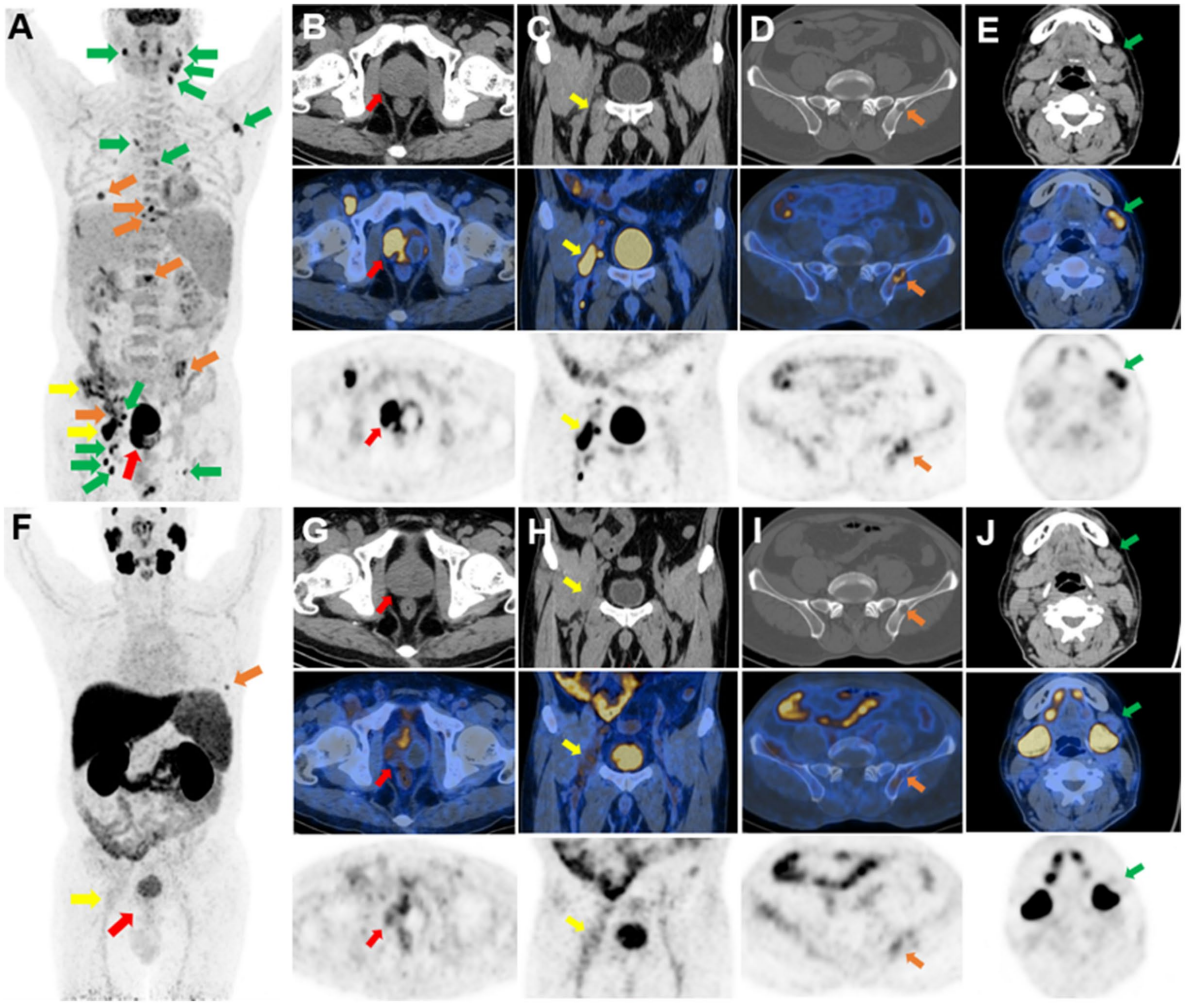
^18^F-FDG and ^18^F-PSMA PET/CT imaging. Multiple FDG-avid lesions (A) on PET MIP images. An unevenly increased FDG uptake on the right side of the prostate, with an SUV_max_ of 17.6 (B). A high FDG uptake in a low-density lesion on the right iliopsoas muscle, with an SUV_max_ of 18.6 (C). Destruction of the left iliac bone with an increased FDG uptake (D). Multiple lymph nodes of the left neck with a high FDG uptake (E). Multiple PSMA-avid lesions (F) on PET MIP images. A slightly unevenly increased PSMA uptake in the prostate (G), the low-density lesion of the right iliopsoas muscle (H), bones (I), and lymph nodes (J).
